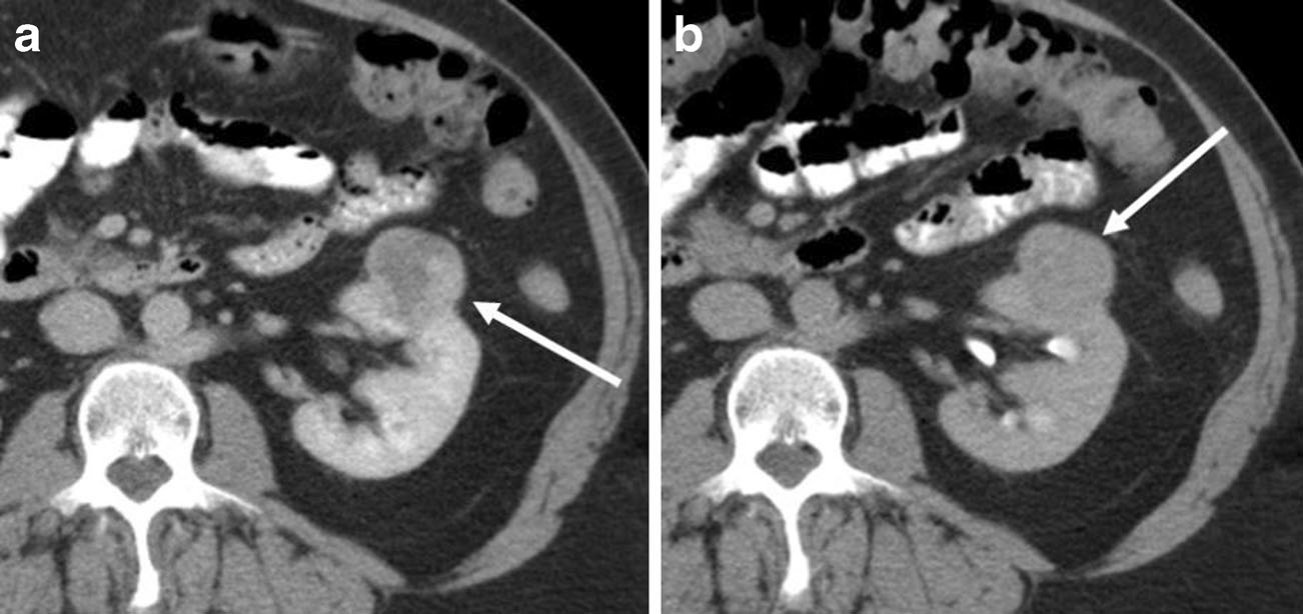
A 51-year-old woman with clear cell RCC of the left kidney, showing homogeneity in the delayed phase. a The nephrographic phase of the axial CE-CT demonstrates a left renal mass consisting of two distinct areas of relative hyper-enhancement (*arrow*) and hypo-enhancement. b In the delayed phase, the mass becomes homogeneous (*arrow*)
